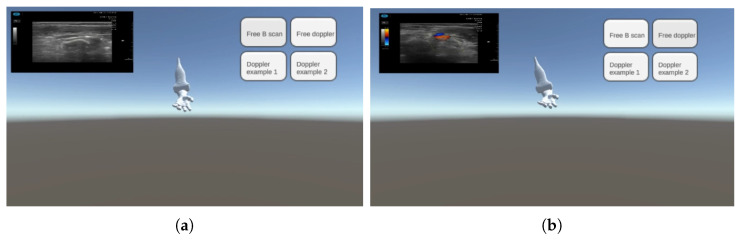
Screenshot of the Current System when training in B-Mode (a) and in Doppler mode (b).
